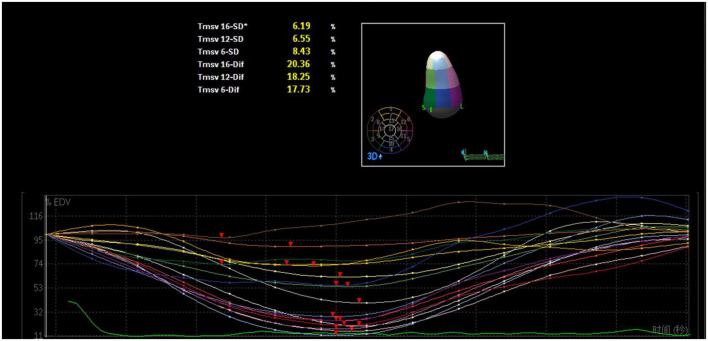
Three-dimensional echocardiography assessment of left ventricular dyssynchrony of a patient with intraventricular dyssynchrony. The time-volume curves are not consistent and lagged, and the disordered time-volume curves signify LV dyssynchrony.
